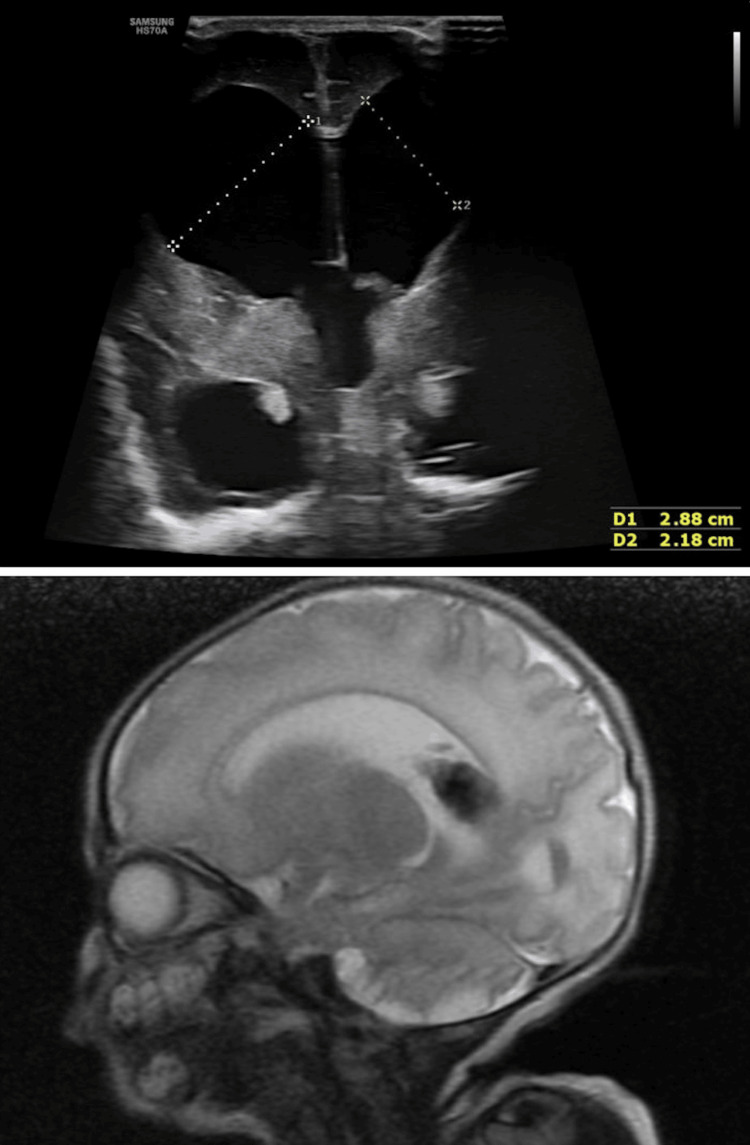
Neurosonography image on the top of a preterm baby shows coronal view with dilatation of bilateral lateral ventricles - suggestive of hydrocephalus. MRI image at the bottom of the same patient correlates with the neurosonography findings. The baby was then taken up for VP shunt insertion. Neurosonography, if done and interpreted properly,y has similar efficacy as MRI and can be used in low-resource settings when MRI is not always available or possible in sick/unstable patients. VP = ventriculo-peritoneal
